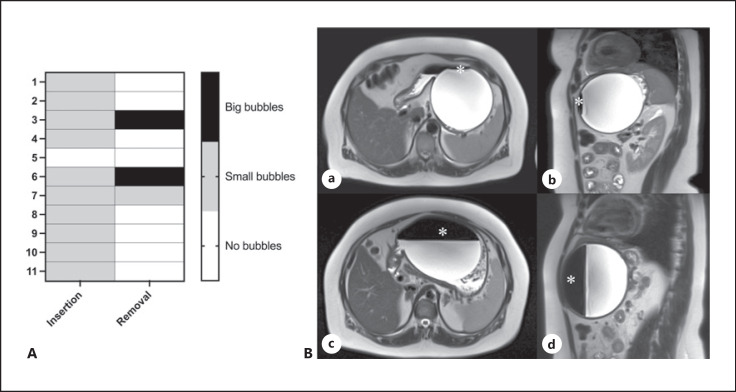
Volume changes of bubbles in the gastric balloon. A The detectable air bubbles showed dynamics at the time of the insertion. In 8 of 11 cases, the air bubbles had disappeared by the time of removal, in 2 cases, the bubbles had increased (patient 3 and 6) and 2 cases showed a stable situation (patient 5 and patient 7 without bubbles). One balloon was passed via naturalis and was thus excluded. B T2-weighted images of a 48-year-old female patient in axial and sagittal plane (a, b, initial scan; c, d, follow-up scan) depicting an enlargement of the gas bubble (*) in the gastric balloon over a period of approximately 6 months. The balloon volume increased from 730 cm^3^ to 1,113 cm^3^.
